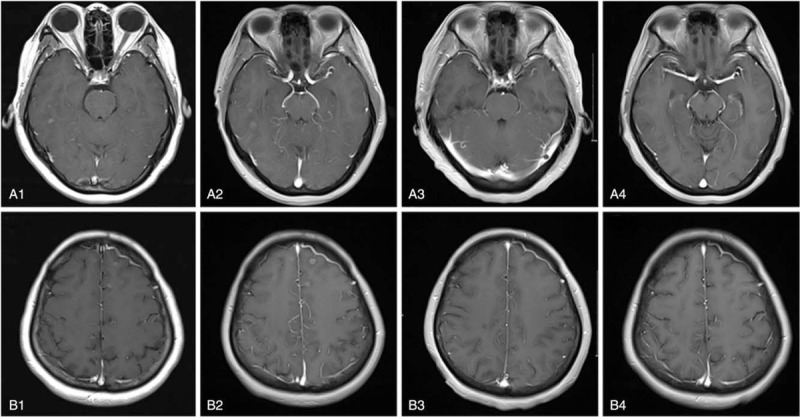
MRI findings in the patient presented with brain metastases. A and B represent a different site. 1, 2, 3, and 4 represent represents different times. A1 and B1. Enhanced, Coronal MRI images demonstrated masses in the cerebral hemisphere on May 12, 2015, and the patient's intracranial lesion progressed. A2 and B2. Enhanced, Coronal MRI images demonstrated masses increase in the size in the cerebral hemisphere on July 29, 2015. The patient's intracranial lesion progressed again. A3 and B3. Enhanced, Coronal MRI images demonstrated no mass in the cerebral t hemisphere on October 20, 2015. The patient's brain metastases disappeared completely. A4 and B4. Enhanced, Coronal MRI images demonstrated no recurrence of brain metastases on November 13, 2018 (39 mo after TMZ). MRI = magnetic resonance imaging, TMZ = temozolomide.
